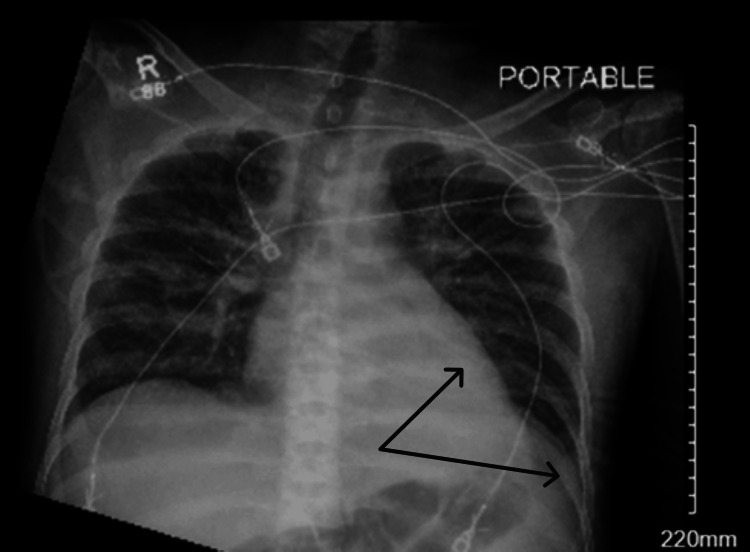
Chest X-ray showing new left lower lobe and retrocardiac opacity
